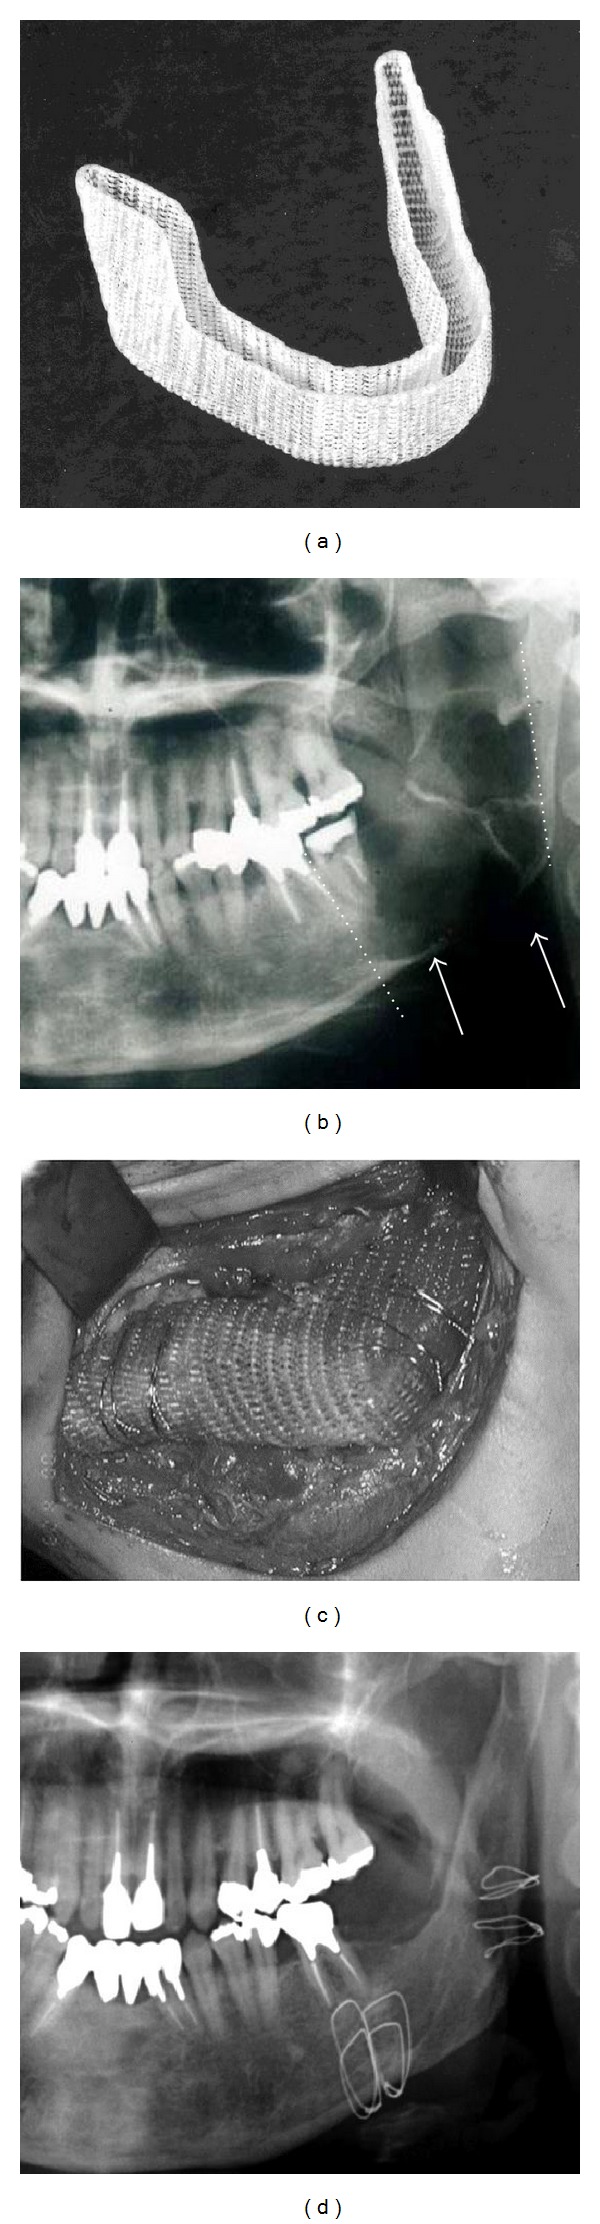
Regeneration of the jaw using PLLA mesh and PCBM [163]. (a) PLLA mesh tray and (b) keratocystic odontogenic tumor of the left mandible, preoperative panoramic X-ray. Multilocular radiolucent area (arrow) and segmental resection line (dotted line). (c) Reconstruction using the PLLA mesh tray and PCBM and (d) X-ray image 1 year and 586 months after the reconstructive surgery. Formation of the matured regenerated bone and mandibular canal.
